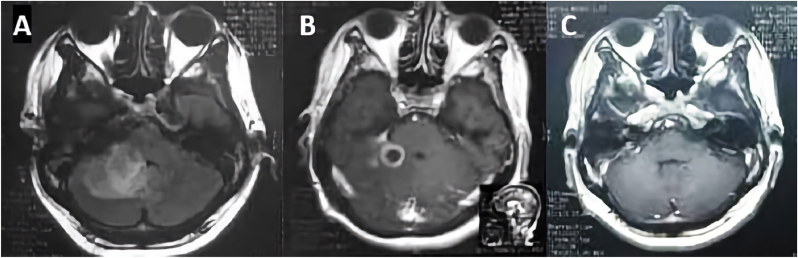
Case 2. A Initial head MRI with contrast. B CT head scan at the fourth week of antibiotic administration. C MRI at the sixth week of antibiotic administration.
